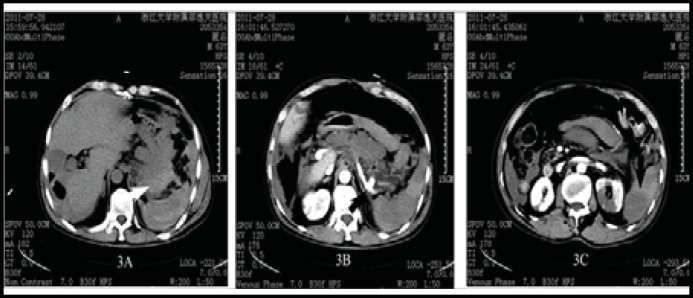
CT demonstrated intraabdominal bleeding in the lesser omental sac (3A), splenic artery aneurysm (black arrow, 3B), and infarction of spleen and left kidney (black arrows, 3C).
